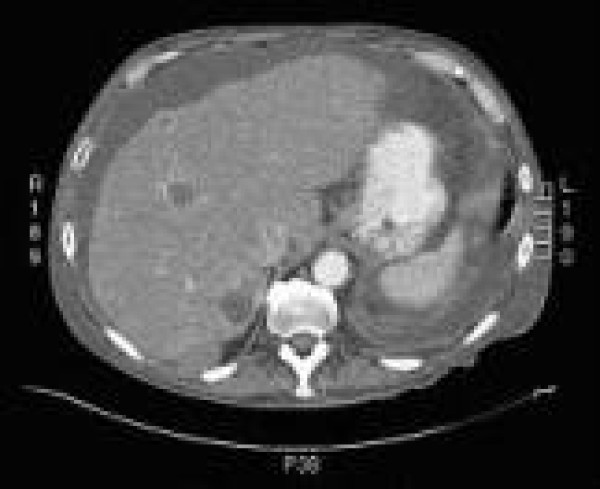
Abdominal CT showing multiple low attenuation lesions with a peripheral rim enhancement of varying sizes in the liver suggestive of metastases or septic emboli.
